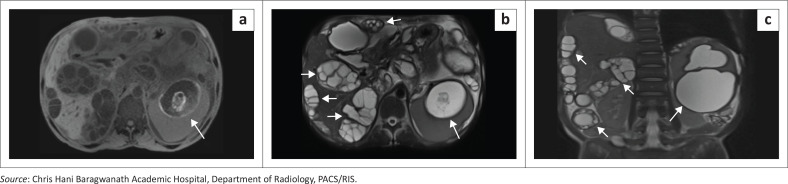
Disseminated hydatid disease in an adult patient. (a) Axial T1-weighted MRI demonstrates diffuse peritoneal hydatidosis with multiple peritoneal lesions. The splenic lesion demonstrates areas of intrinsic T1 hyperintensity and a peripheral hyperintense rim (arrow). (b) Axial T2-weighted HASTE sequence demonstrates multiloculated peritoneal hydatid cysts and a well-circumscribed splenic hydatid cyst with peripheral signal dropout corresponding to a calcified rim and internal multiseptated daughter cysts (arrow). (c) Coronal T2-weighted HASTE image confirms the splenic origin of the dominant lesion and demonstrates additional peritoneal and hepatic hydatid lesions (arrows).
